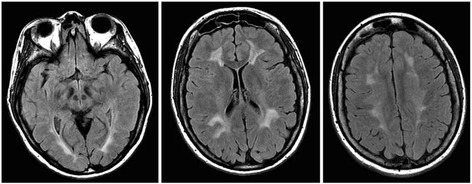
Brain MRI showing white matter changes (patient n°2). Periventricular and subcortical hyper signals with sparing of the U fibers (Axial T2 FLAIR)
